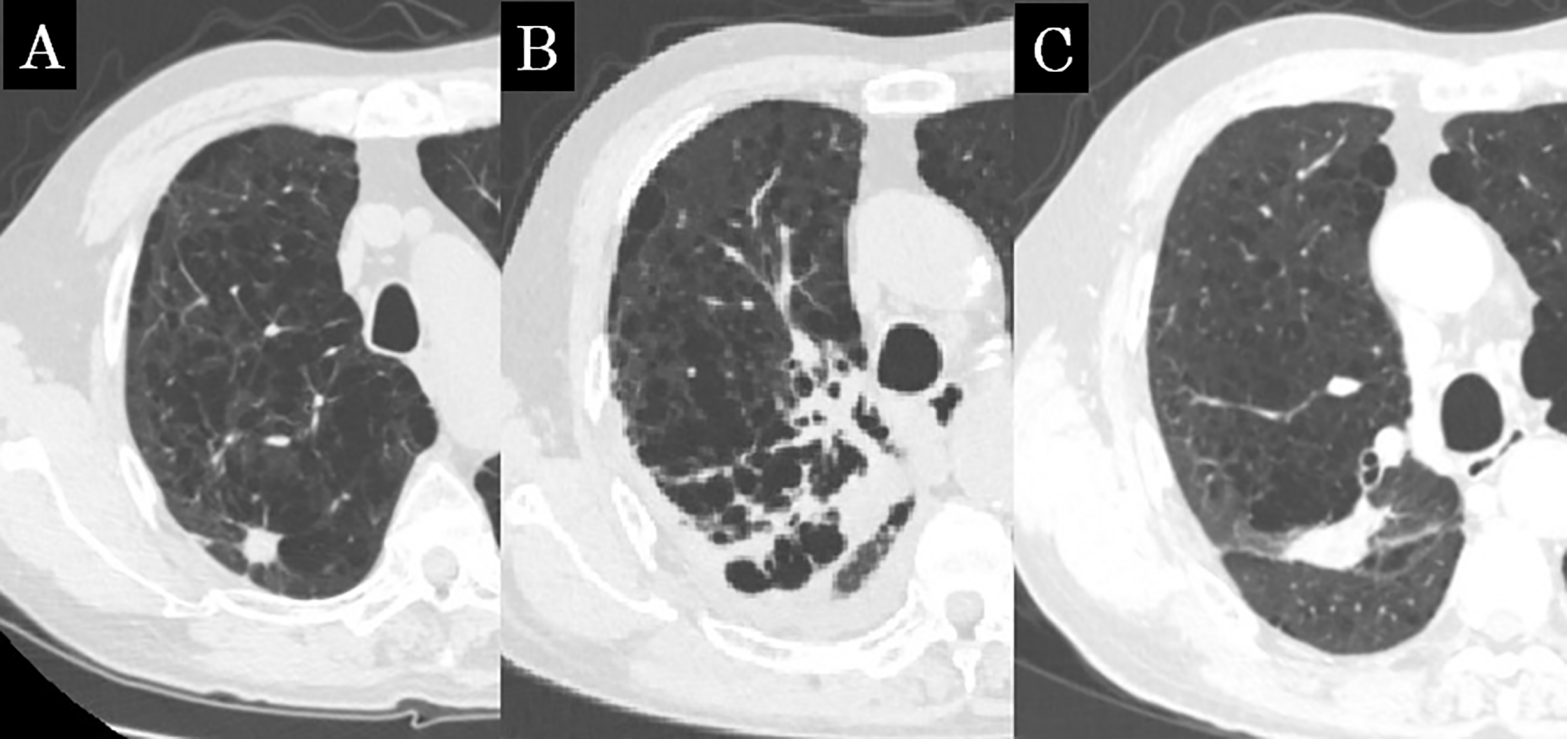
Concordance CT appearance, case 2. (a) Before SBRT, the lung tumor was located in the right upper lobe. (b) The consolidation expands beyond the high-dose region; this shadow was diagnosed as diffuse consolidation at 5 months after SBRT. (c) The shadow shrank, and only a linear opacity remained. This shadow was diagnosed as a scar-like pattern at 20 months after SBRT.
